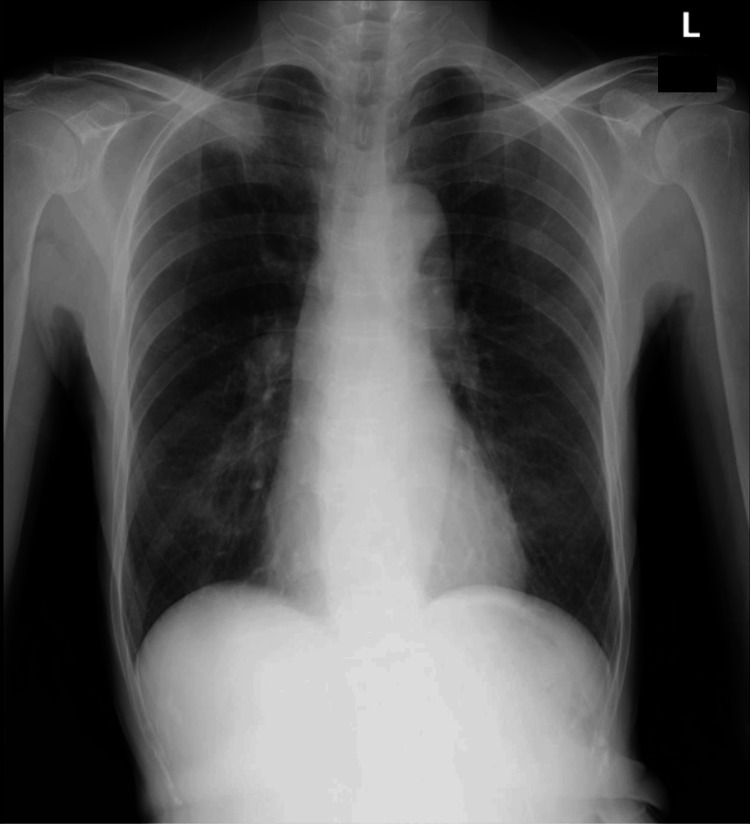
Results of preoperative chest X-ray. The preoperative chest X-ray findings did not clearly indicate tracheal stenosis.
